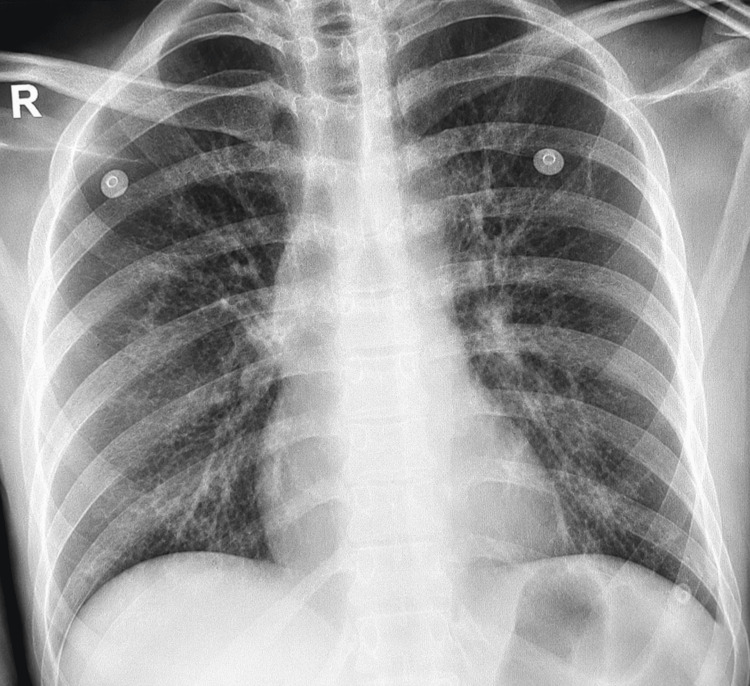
Follow-up chest X-ray showing interval resolution of bilateral opacities with clear lung fields
